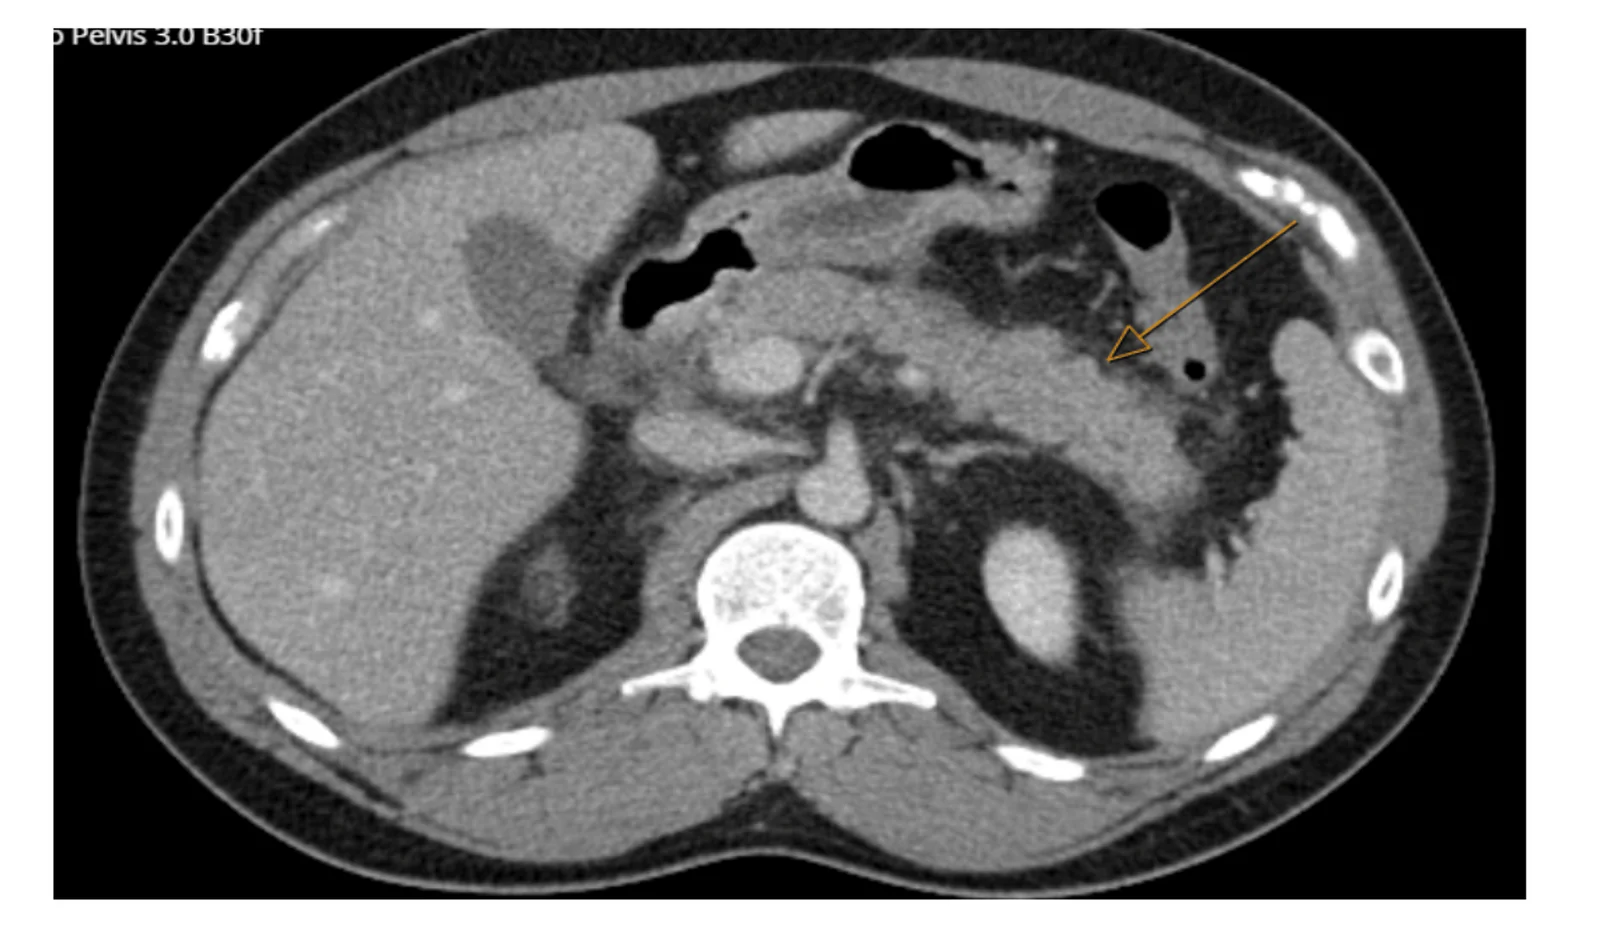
Axial contrast-enhanced CT of the abdomen and pelvis showing an enlarged, inflamed pancreas with surrounding fat stranding, consistent with acute pancreatitis (arrow)
